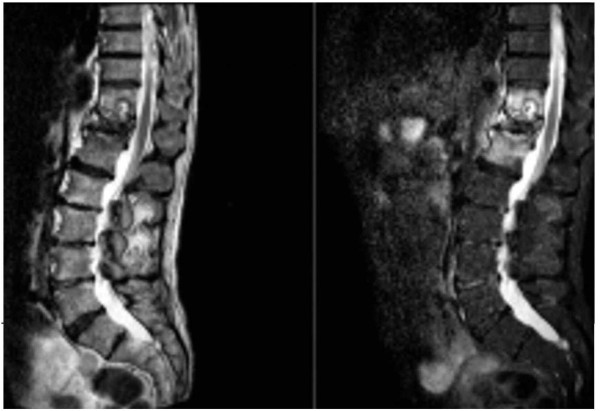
T2-weighted magnetic resonance imaging scan and short tau inversion recovery sequence sagittal cuts showing further effusion of T11.
